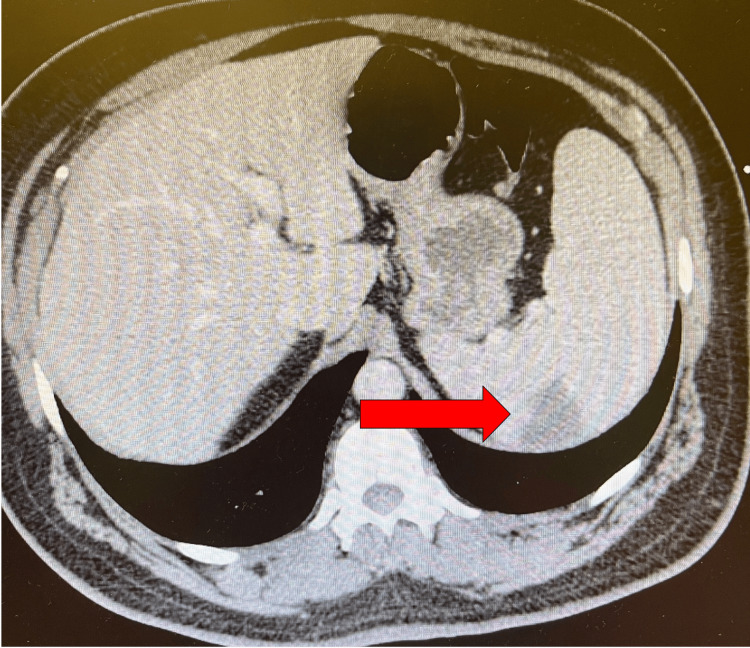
Splenic infarct seen on CT imaging
